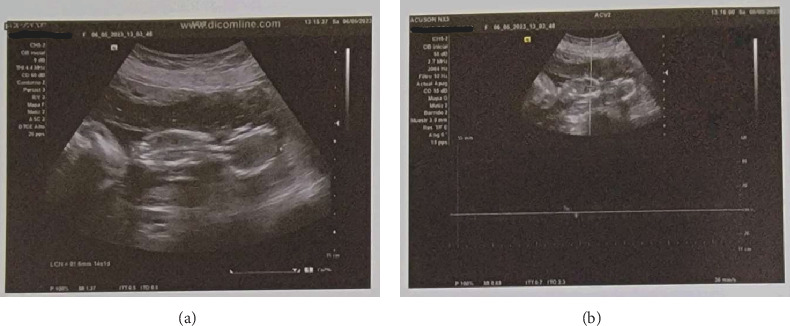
Antenatal ultrasound image showing one twin (b) and absence of fetal heart rate using m-mode (a).
